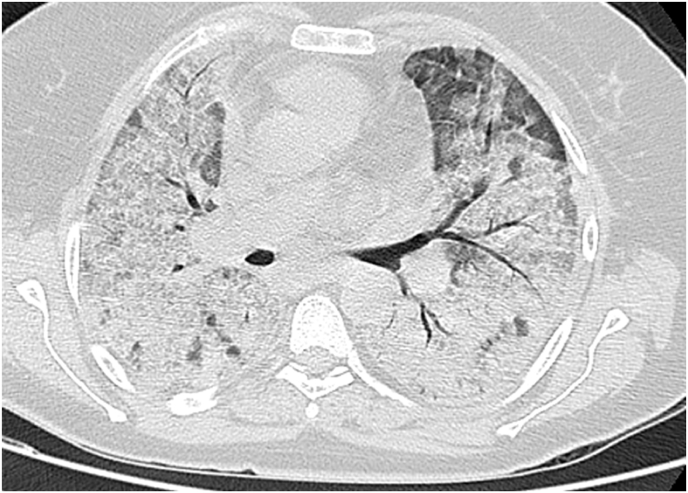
CT image showing involvement of the lungs superior than 75%, classified CORADS 6.
